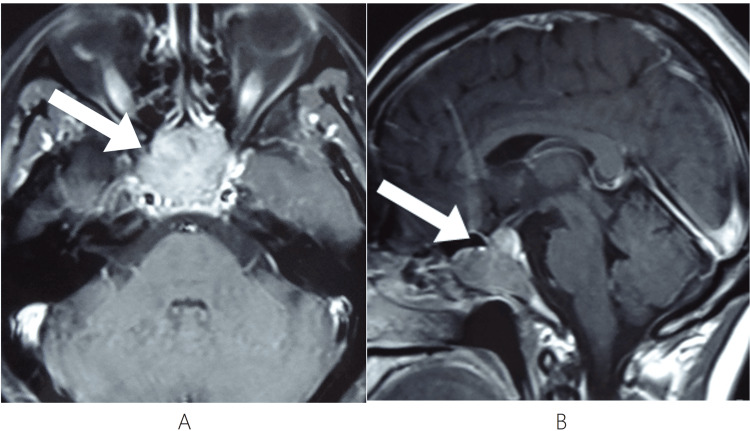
Pre-surgery Baseline - March 2014 A mass with an abnormal signal is seen in the sellar region, compressing the optic chiasm superiorly. (A) Axial T1-weighted post-contrast image shows a sellar mass with heterogeneous mild-to-moderate enhancement (arrow). (B) Sagittal T1-weighted post-contrast image shows the lesion with heterogeneous mild-to-moderate enhancement (arrow).
